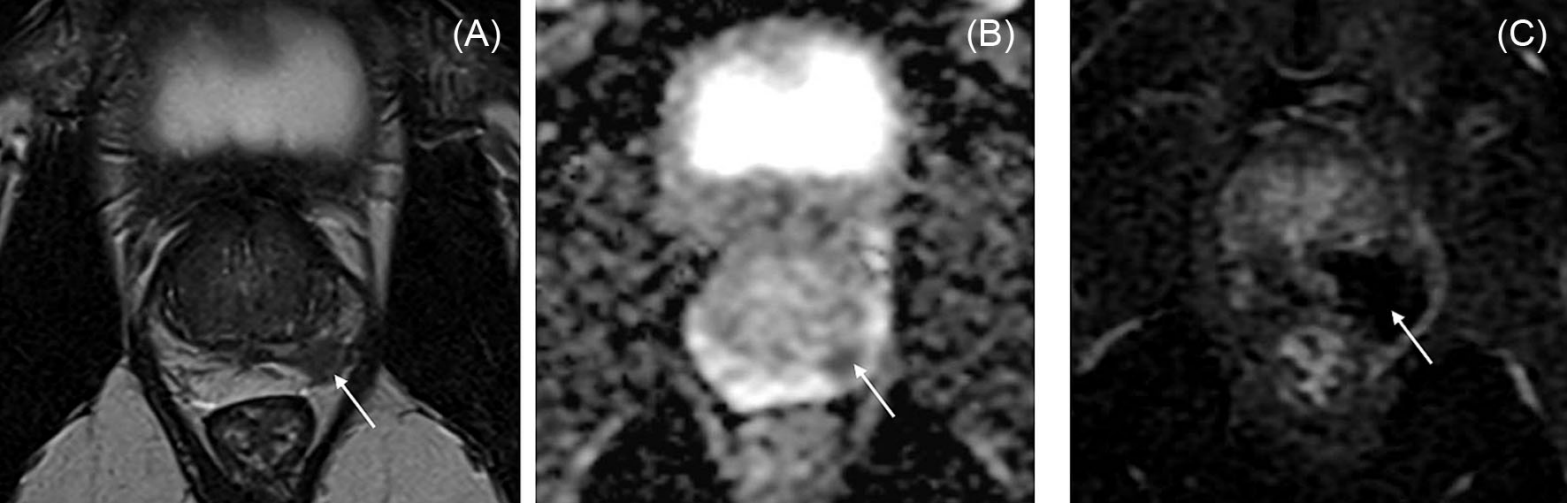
(A) MRI T2 and (B) ADC image showing an index tumour of 12 mm of largest axis (white arrow), treated with two ablations of 4 min (treatment margin set à 4 mm). (C) Dynamic MRI performed 7 days after treatment shows an avascular area (white arrow) covering entirely the index tumour.
